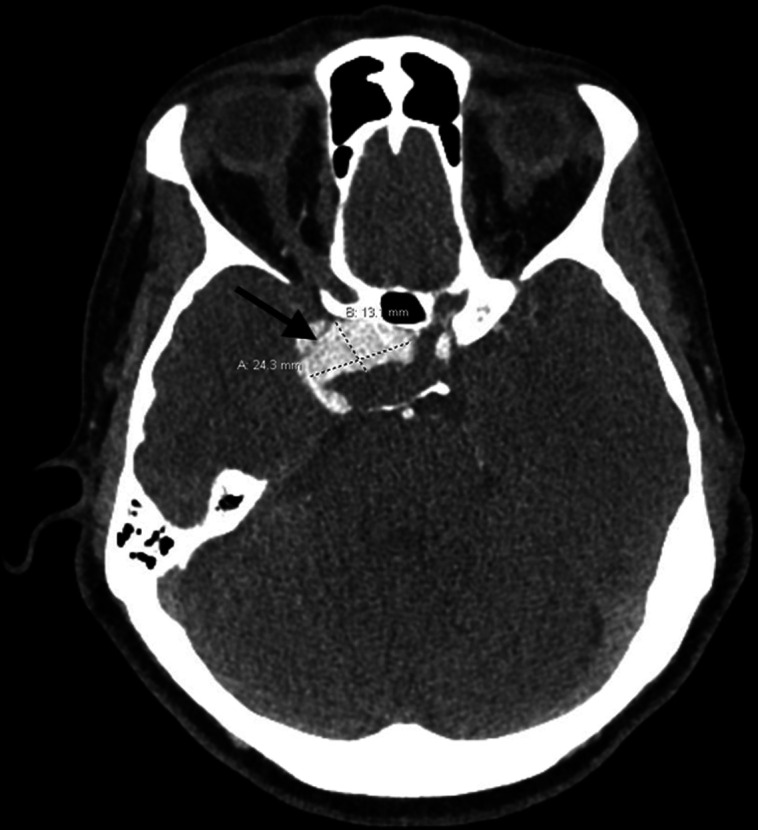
Computed tomography angiography of the brain revealed a large mass involving the pituitary fossa and right cavernous sinus concerning for a large, partially thrombosed aneurysm arising from the distal cavernous portion of right internal carotid (black arrow).
